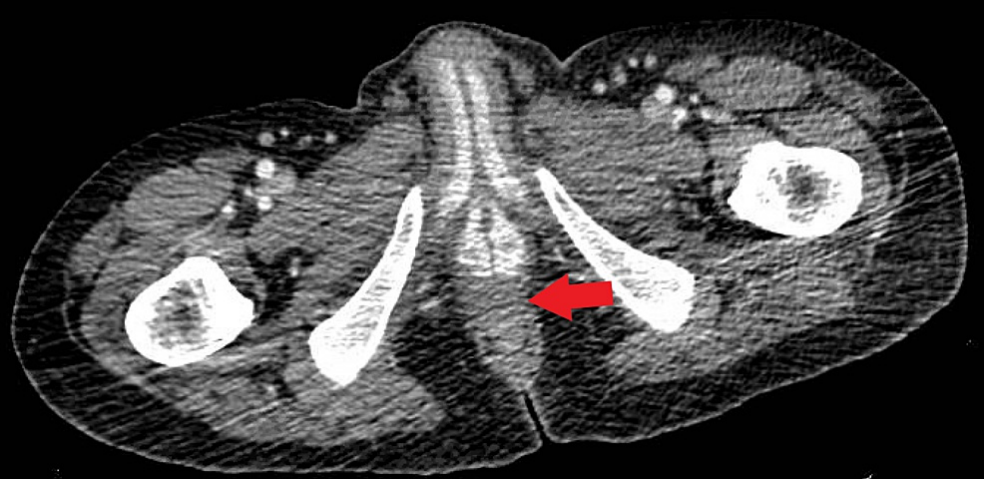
Perirectal fluid shown in CT of the abdomen and pelvis
